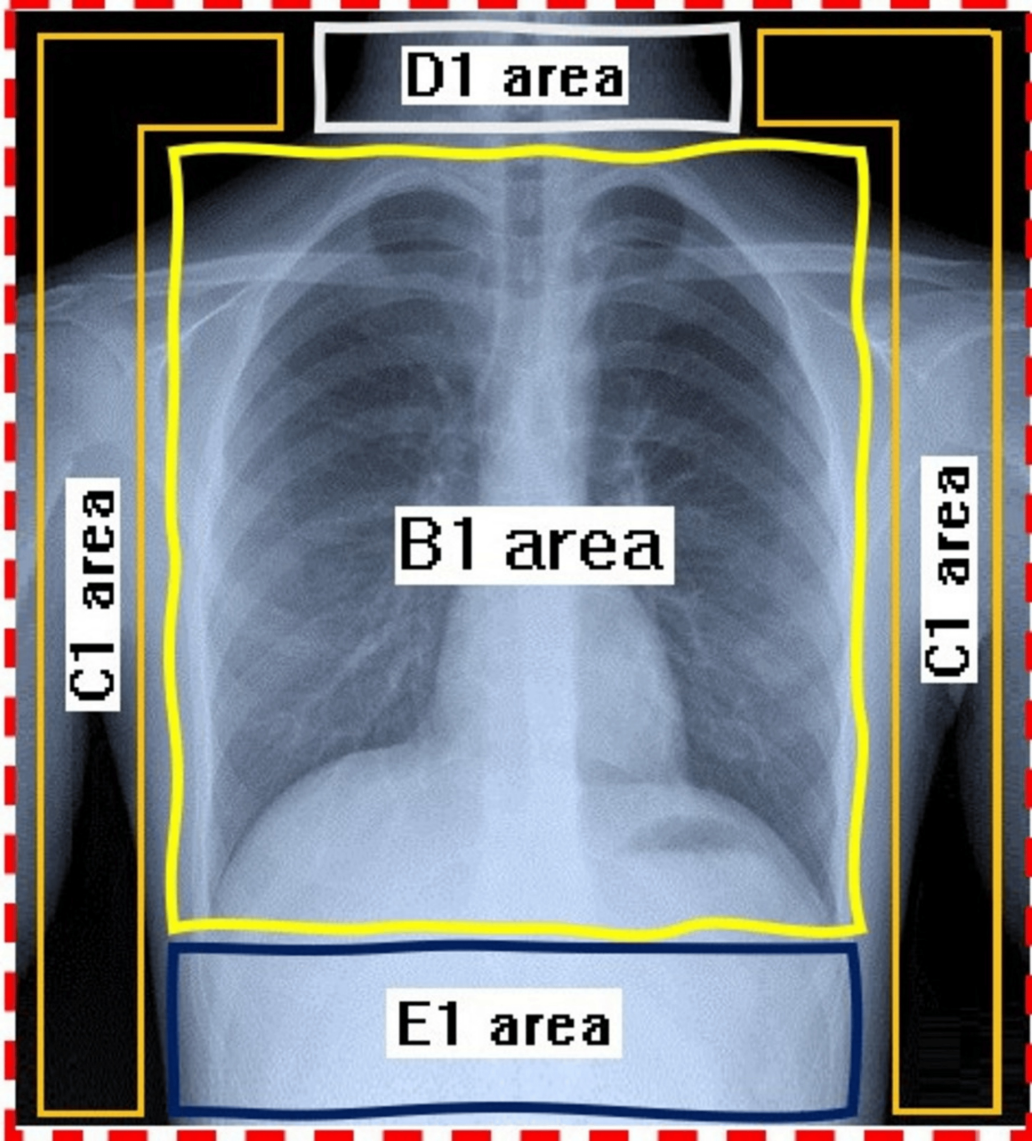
The determined collimated areas of a chest radiograph image: A1 (the red dashed line; field of view area), B1 (the yellow line; field of interest), C1 (the orange line; the margin area), D1 (the white line; the neck area), and E1 (the dark blue line; the abdominal area).
